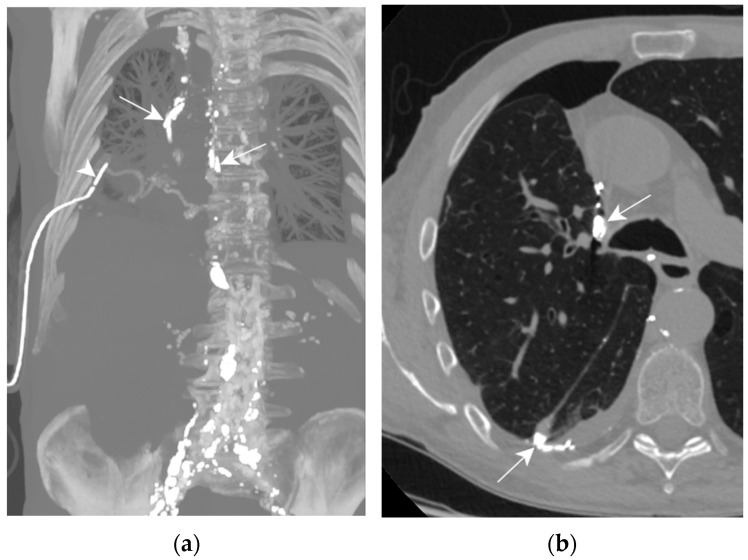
Patient D. The axial CT plane through the upper pulmonary lobes with magnification on the right hemithorax (b); the maximal-intensity projection of the CT scan reconstructed in the coronal plane (a). (a,b) The presence of Lipiodol in a right pleural effusion along the mediastinum and in its declive portion (white arrows). Thoracic drainage with a pleural catheter (white arrowhead) is shown.
